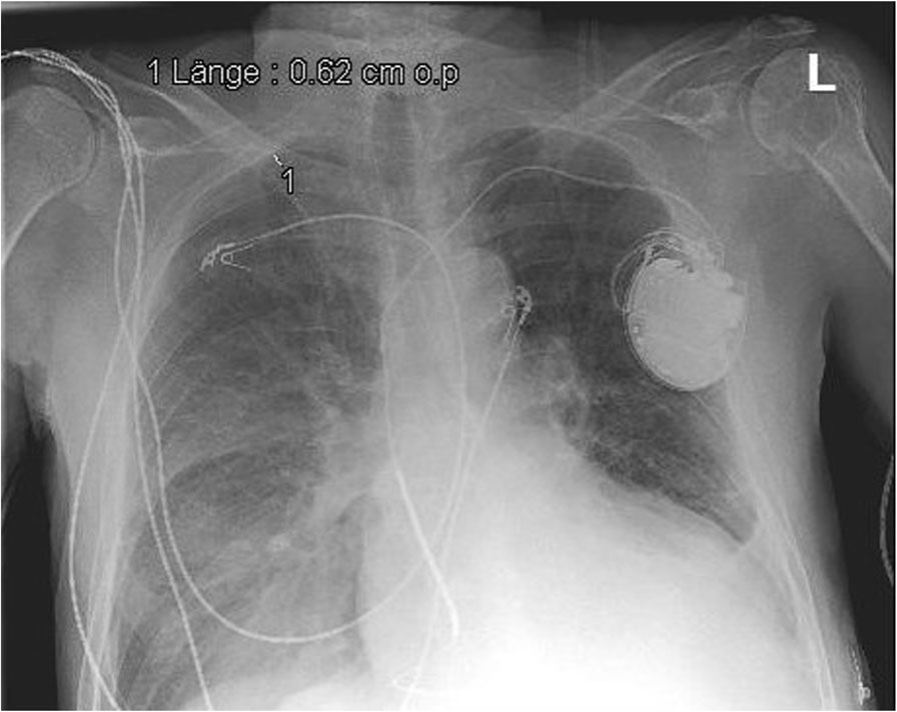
X-Ray 03/27/2020, lunge after draining 1.1 l pulmonal fluids
